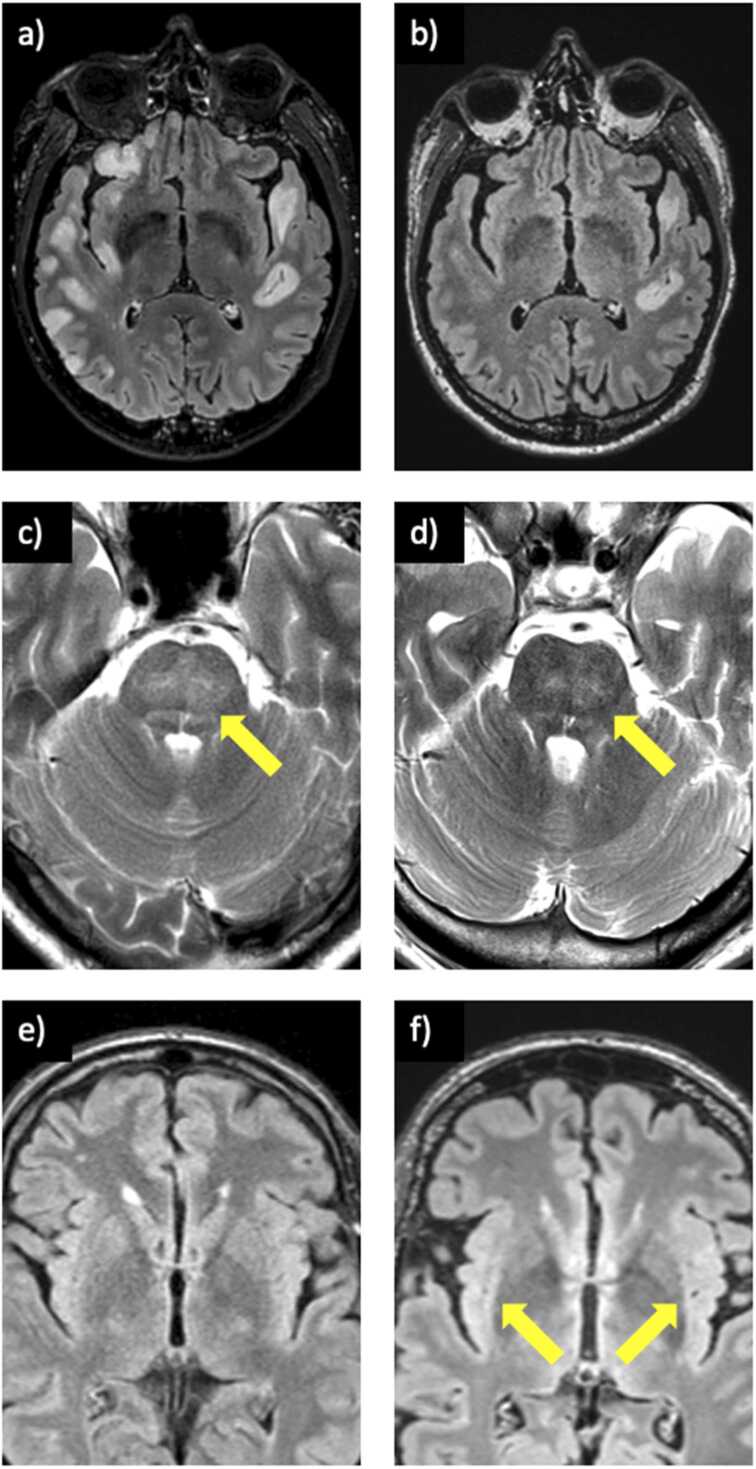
Further examples of autoimmune encephalitis with follow up imaging. (a) A patient with anti-GABAaR encephalitis demonstrating multifocal, asymmetrical FLAIR hyperintense lesions involving the cortex and subcortical white matter on initial MRI; (b) marked improvement on MRI at day 156 after treatment with corticosteroids and plasma exchange. (c) A patient with anti-Hu encephalitis demonstrating signal change within the pons (arrow); (d) follow-up MRI on day 141 after treatment with plasma exchange and IVIG showed improvement but not complete resolution of findings (arrow). (e) A patient with anti-CASPR2 encephalitis with a bilaterally normal-appearing claustrum on initial MRI; (f) MRI performed four years later reveals new signal change in the claustrum bilaterally (arrows) despite treatment with corticosteroids and IVIG.
